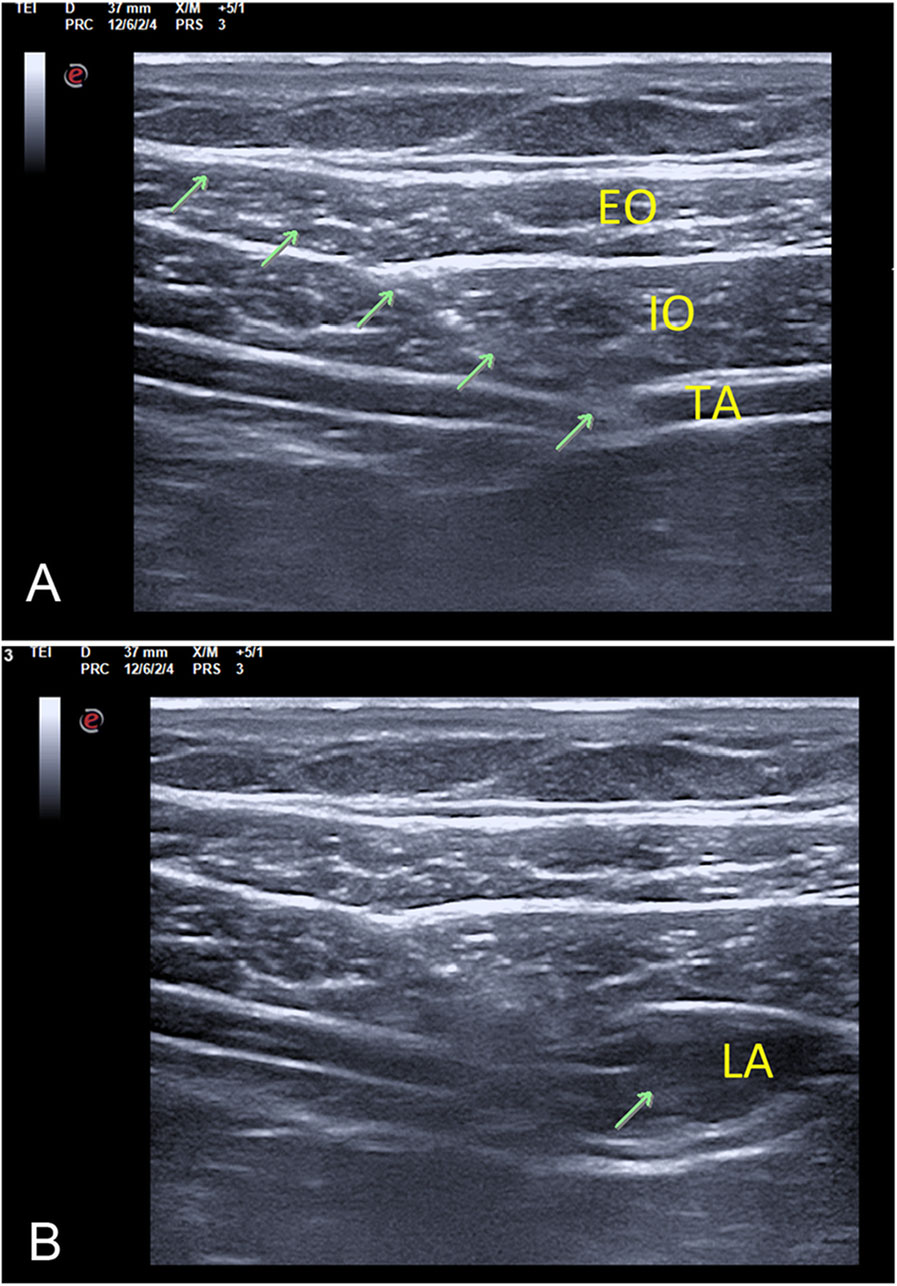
Sonography of the lateral TAP block, indicating the ultrasound anatomical structure (a) and the spread of local anaesthetic (b). The green arrow indicates the needle trajectory; EO: external oblique; IO: internal oblique; TA: transversus abdominis; LA: local anaesthetic
